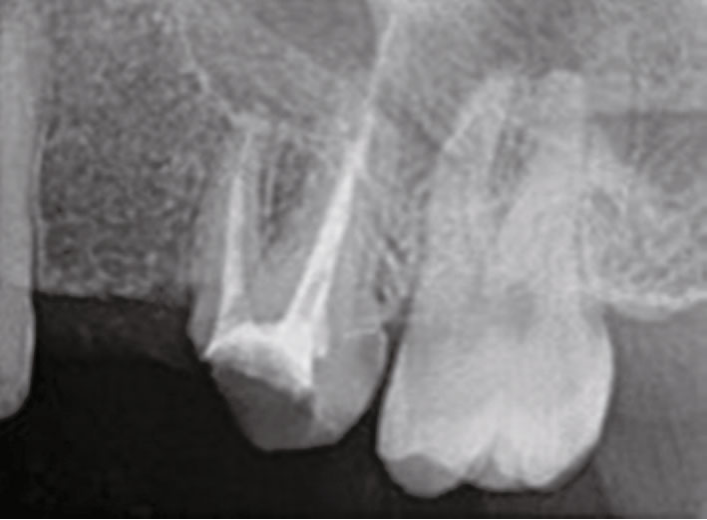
Preoperative radiograph. The intraoral periapical radiograph showed radiolucency at the root apex and overextended gutta-percha through a perforation in the apical part of one of the three roots of the maxillary left first molar.
